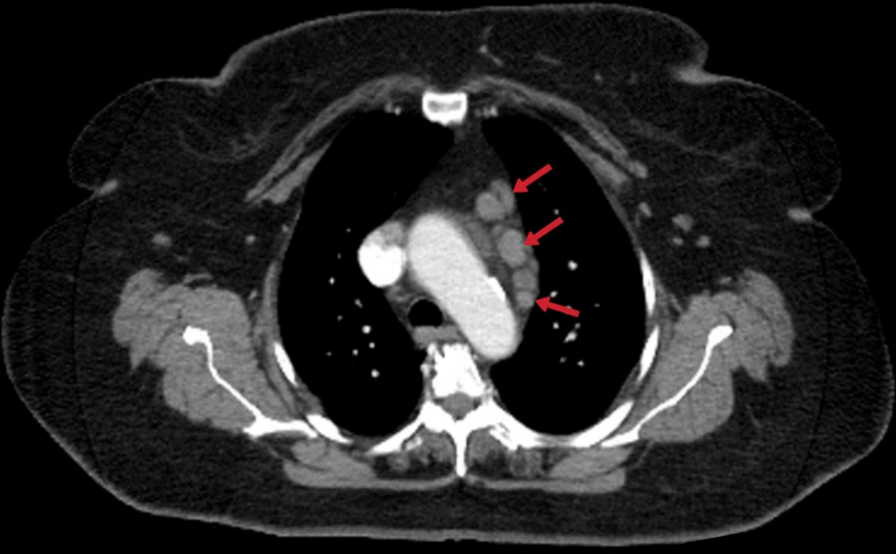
Contrast-enhanced thorax CT image of a 70-year-old female. Multiple mediastinal lymphadenopathies are noted in the prevascular region (red arrows)
